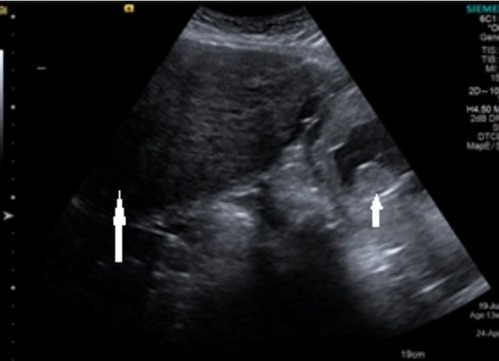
Ultrsonographic picture showing intrauterine fetus (short arrow) with large mass (long arrow) in relation to fundus
